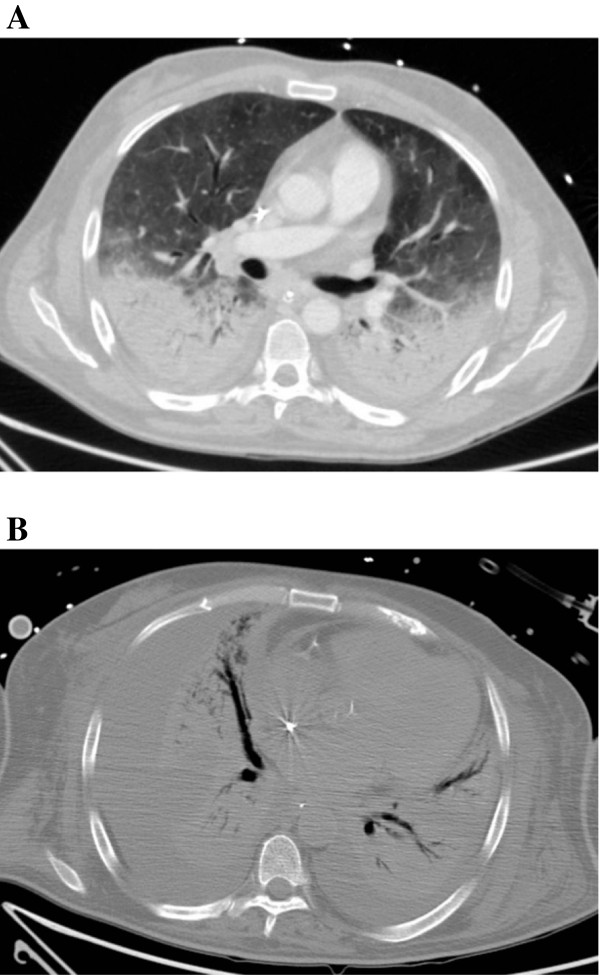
Thoracic computed tomography (CT) before ECMO implantation (A) and after 40 days of ECMO support (B) in Patient 1. A. Bilateral parenchymal consolidation in gravity-dependent areas and ground-glass-appearing opacities of lung parenchyma, compatible with severe ARDS. B. Extensive parenchymal consolidation and bilateral pleural effusions with associated compression atelectasis after 40 days of VV-ECMO.
